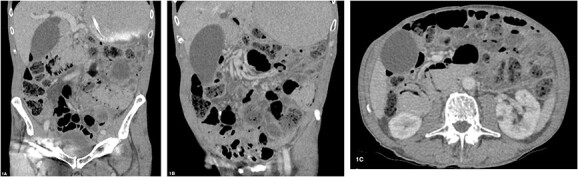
(A–C) Abdominal CT scan with oral and intravenous contrast revealing PI with gastric and proximal small bowel dilatation, without evidence of perforation or ischaemia.
